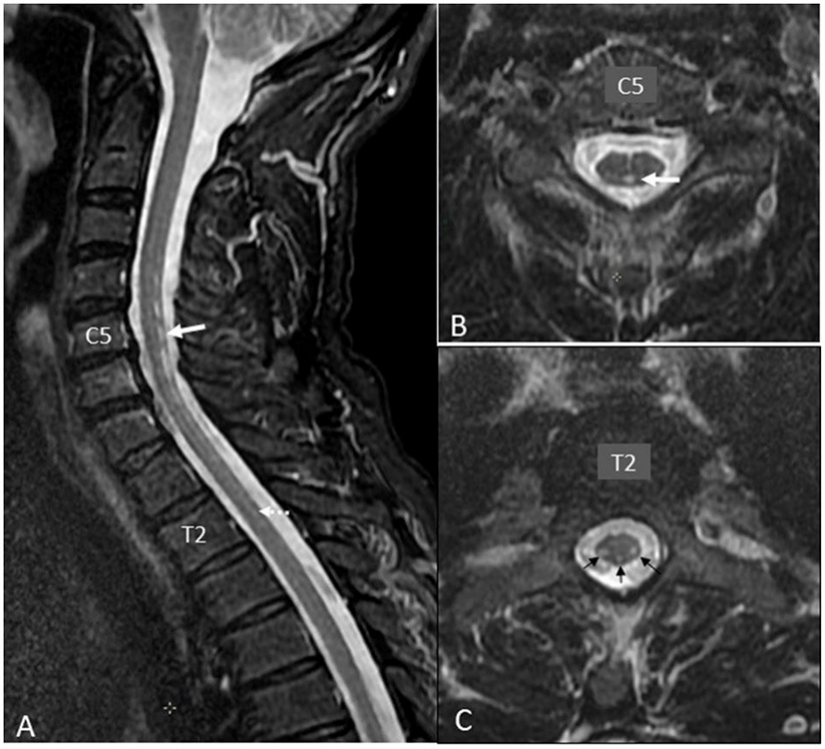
Spinal MR imaging (MRI) workup. (A) Mid-sagittal T2-weighted view from C0 to T5 showing linear hypersignal intensity within posterior columns prominently at the level of C5–C6 (white arrow). At the thoracic level, only faint signal intensity changes were suspected (dotted arrow at T2 level). (B) Axial transverse T2-weighted view at C5 level showing the posterior mid-sagittal focus of abnormal hypersignal intensity (arrow) reflecting elective tissue damage to the posterior columns. (C) Axial transverse T2-weighted view at T2 level showing decrease in size of tissue damage within posterior columns (middle arrow), but the appearance of abnormal hypersignal intensity within both crossed cortico-spinal tracts (lateral arrows), which were mostly outside slice location on mid-sagittal T2 view (1A).
